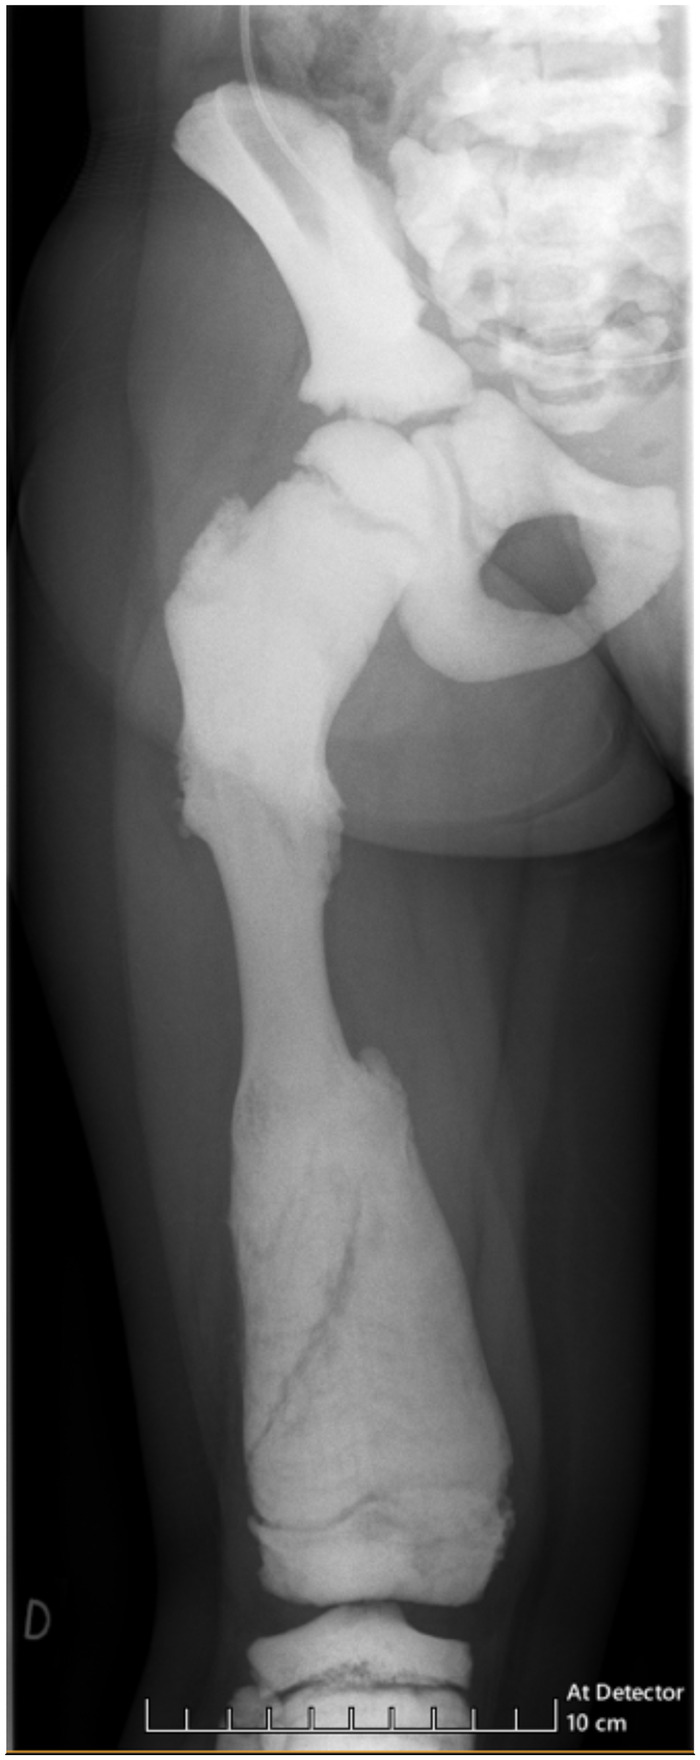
AP radiograph of the right femur of a 10-year-old patient with ADO II. It reveals the typical flask-shaped deformity of the distal femoral metaphysis.
